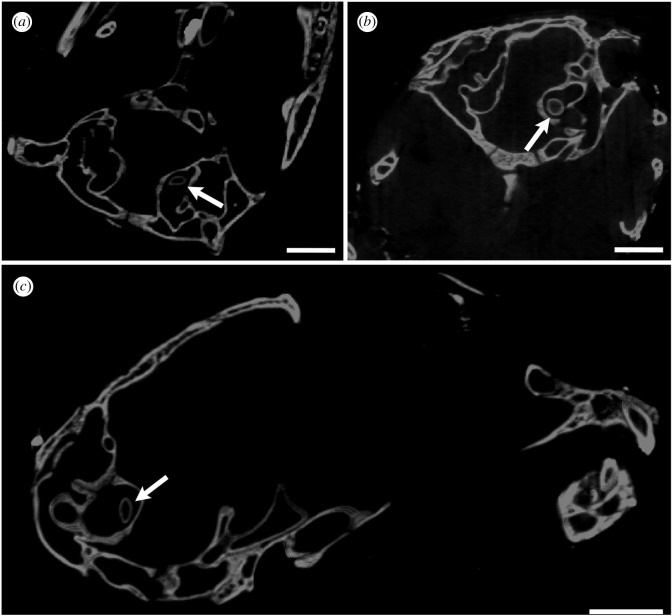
CT slices of *Melanosuchus niger* (NMS.Z.1859.13.804) with the otolith shown in the vestibule of the bony labyrinth (white arrow). (*a*) axial, (*b*) coronal, (*c*), sagittal views. Scale bars equal 5 mm.
